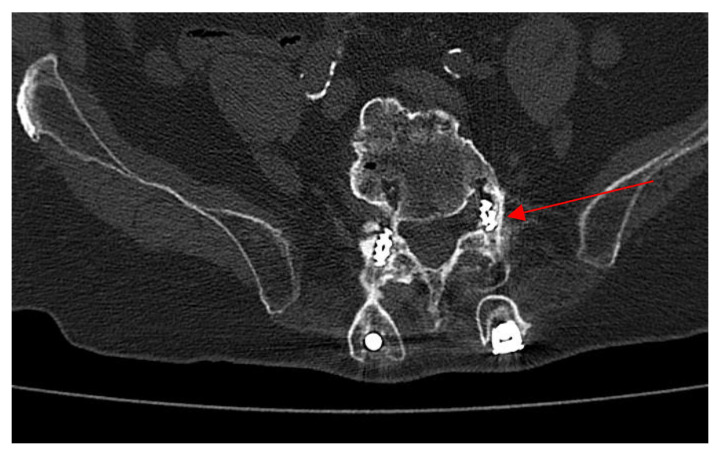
Lumbosacral computed tomography scan, the left L5 pedicle screw obliterates the ipsilateral preforaminal recess (red arrow).
